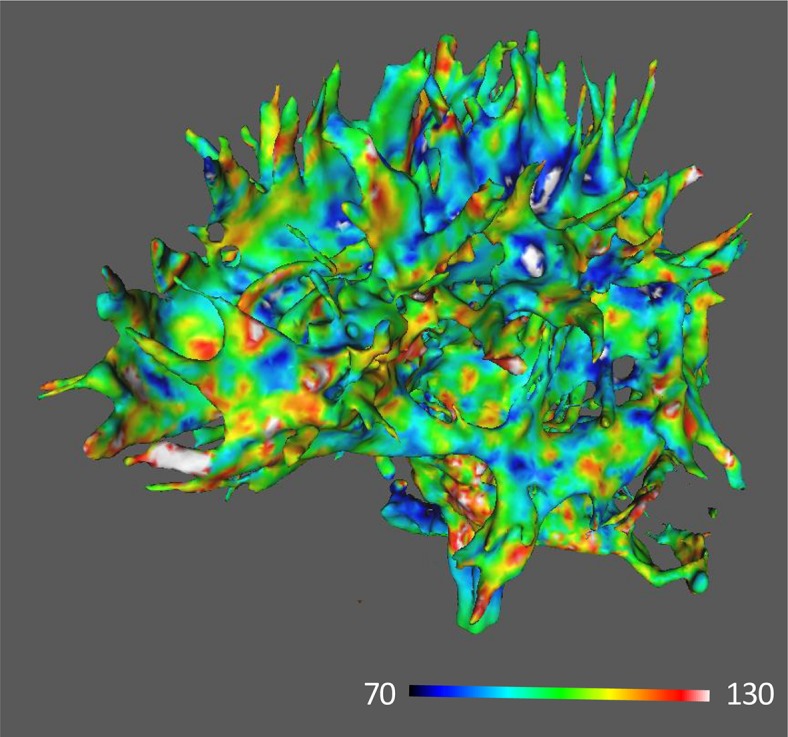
MRI image of the lowest FA-BHQ score.
